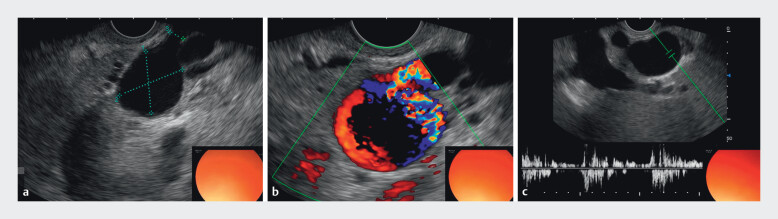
EUS images showing a 2.7 cm × 1.8 cm SAA from the distal splenic artery with bidirectional “to-and-fro” blood flow on Doppler.
